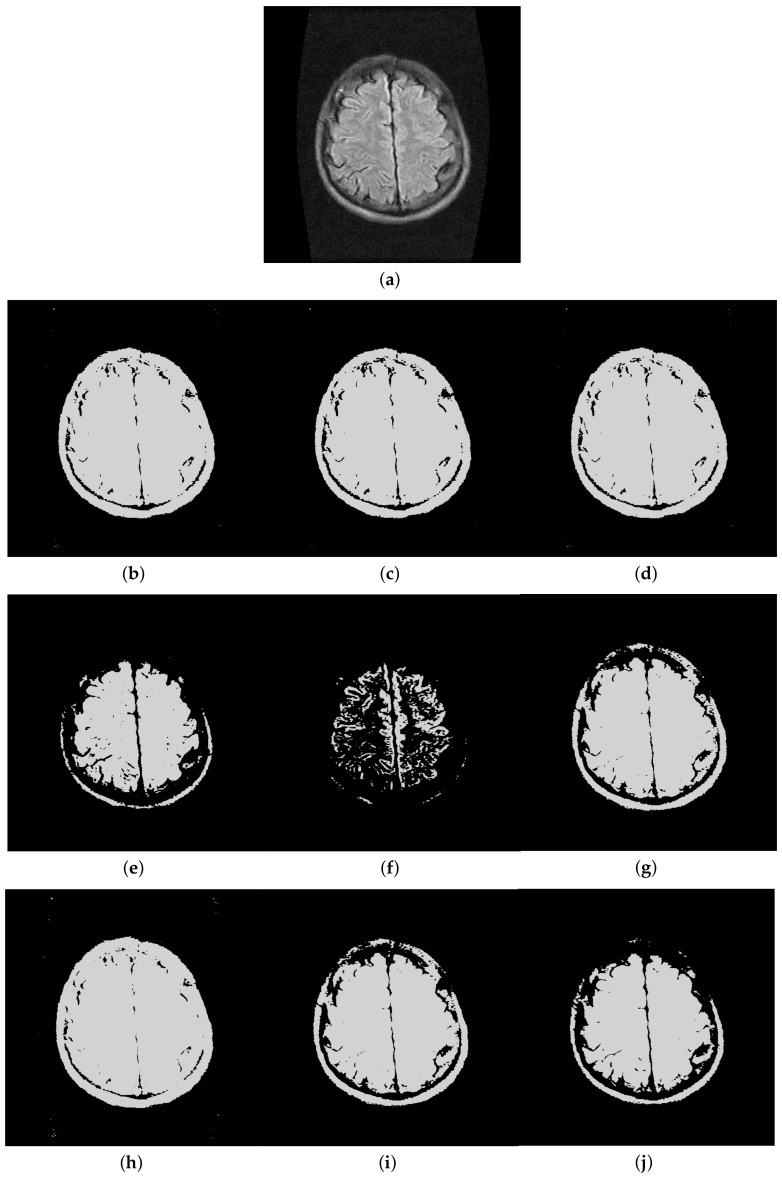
Segmented results of Magnetic Resonance Imaging (MRI) image: (a) original image; (b) 1D Fisher; (c) 1D cross entropy; (d) 1D maximum entropy; (e) 1D Tsallis entropy; (f) fuzzy entropy; (g) 2D Fisher; (h) 2D cross entropy; (i) 2D maximum entropy; (j) proposed method.
